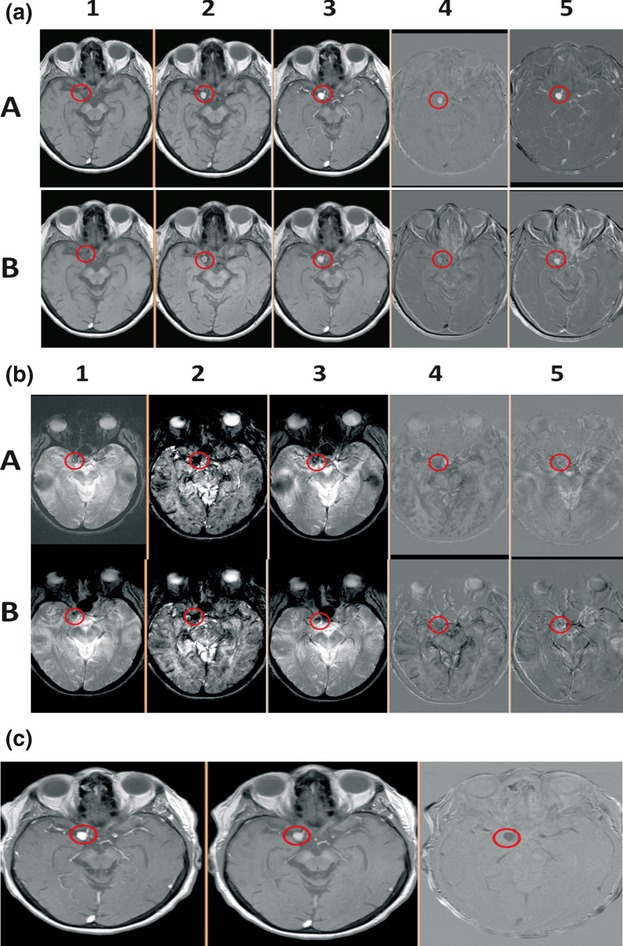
Right posterior carotid wall aneurysm from a 69‐year‐old female patient. (a) T1 spin‐echo sequence. Images A1 to A5 are baseline images (ie, before aspirin treatment). A1, Before ferumoxytol infusion. A2, Immediately after ferumoxytol infusion. A3, Seventy‐two hours after ferumoxytol infusion. A4, A2 minus A1. A5, A3 minus A1. Images B1 to B5 were obtained after 3 months of aspirin treatment. B1, Before ferumoxytol infusion. B2, Immediately after ferumoxytol infusion. B3, Seventy‐two hours after ferumoxytol infusion. B4, B2 minus B1. B5, B3 minus B1. (b) T2* sequence. Images A1 to A5 are baseline images (ie, before aspirin treatment). A1, Before ferumoxytol infusion. A2, Immediately after ferumoxytol infusion. A3, Seventy‐two hours after ferumoxytol infusion. A4, A2 minus A1. A5, A3 minus A1. Images B1 to B5 were obtained after 3 months of aspirin treatment. B1, Before ferumoxytol infusion. B2, Immediately after ferumoxytol infusion. B3, 72 hours after ferumoxytol infusion. B4, B2 minus B1. B5, B3 minus B1. (c) T1 spin‐echo sequence. Left, baseline image (ie, before aspirin treatment) 72 hours after ferumoxytol infusion; middle, 3‐month follow‐up images (after aspirin treatment) obtained 72 hours following ferumoxytol infusion; right, A2 minus A1. Note the decrease in signal intensity on various magnetic resonance imaging (MRI) sequences after aspirin treatment.
